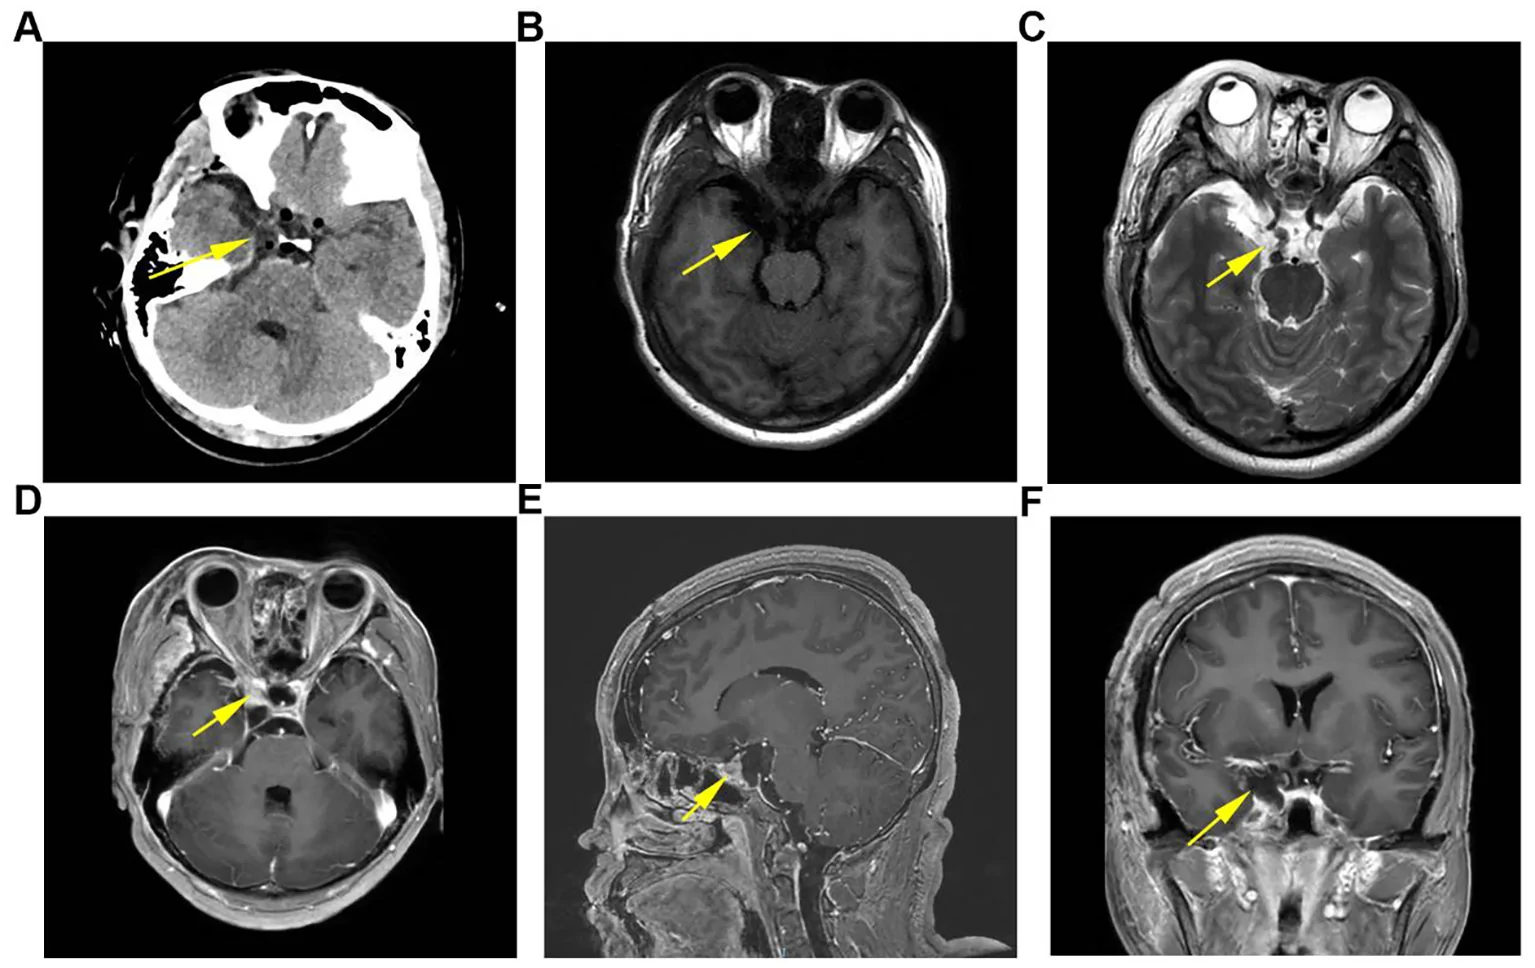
Postoperative imaging. (A) Axial non-contrast CT (brain window) showing no abnormal density at the surgical site. (B) Axial T1WI and (C) axial T2WI demonstrating no definite residual mass. (D) Axial and (E) sagittal CE-T1WI revealing a small residual enhancing focus in the right cavernous sinus (arrows). (F) Coronal CE-T1WI confirming near-total resection.
